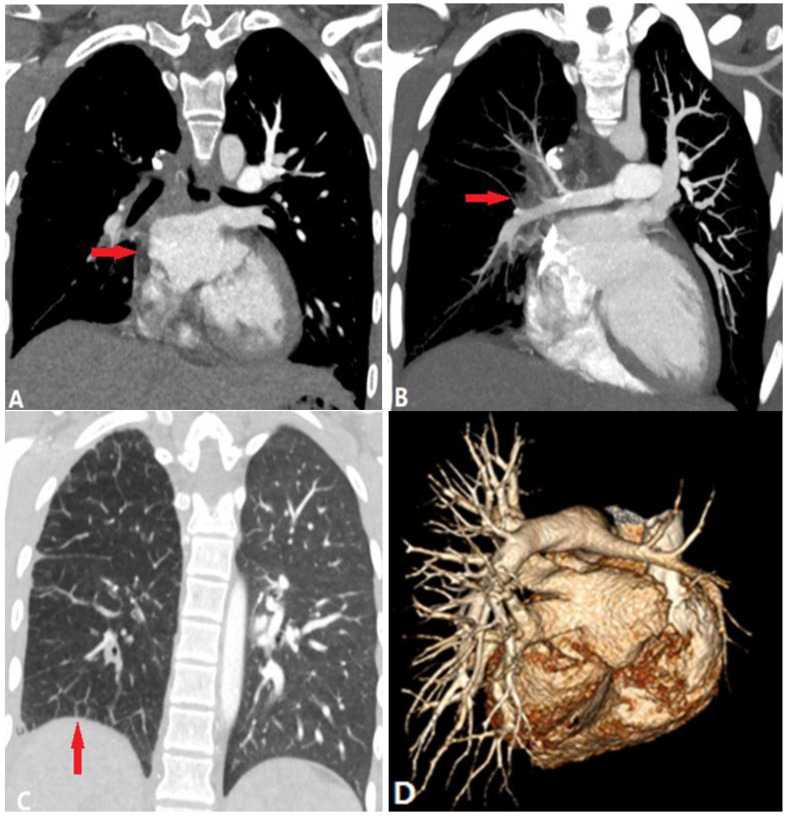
Unilateral pulmonary vein atresia. (A): Coronal CTA shows the absence of right pulmonary veins and outpouchings on the left atrium (arrow). (B,C): MIP and lung windowing coronal CT images show mass-like soft tissue within the right hilum (arrow in (B)), septal lines and bronchial-wall thickening (arrow in (C)), which indicate the engorged lymphatics and collateral venous channels. (D): VR of CTA shows the small caliber of right pulmonary arteries. CTA—computed tomography angiography; MIP—maximum-intensity projection; VR—volume-rendering.
